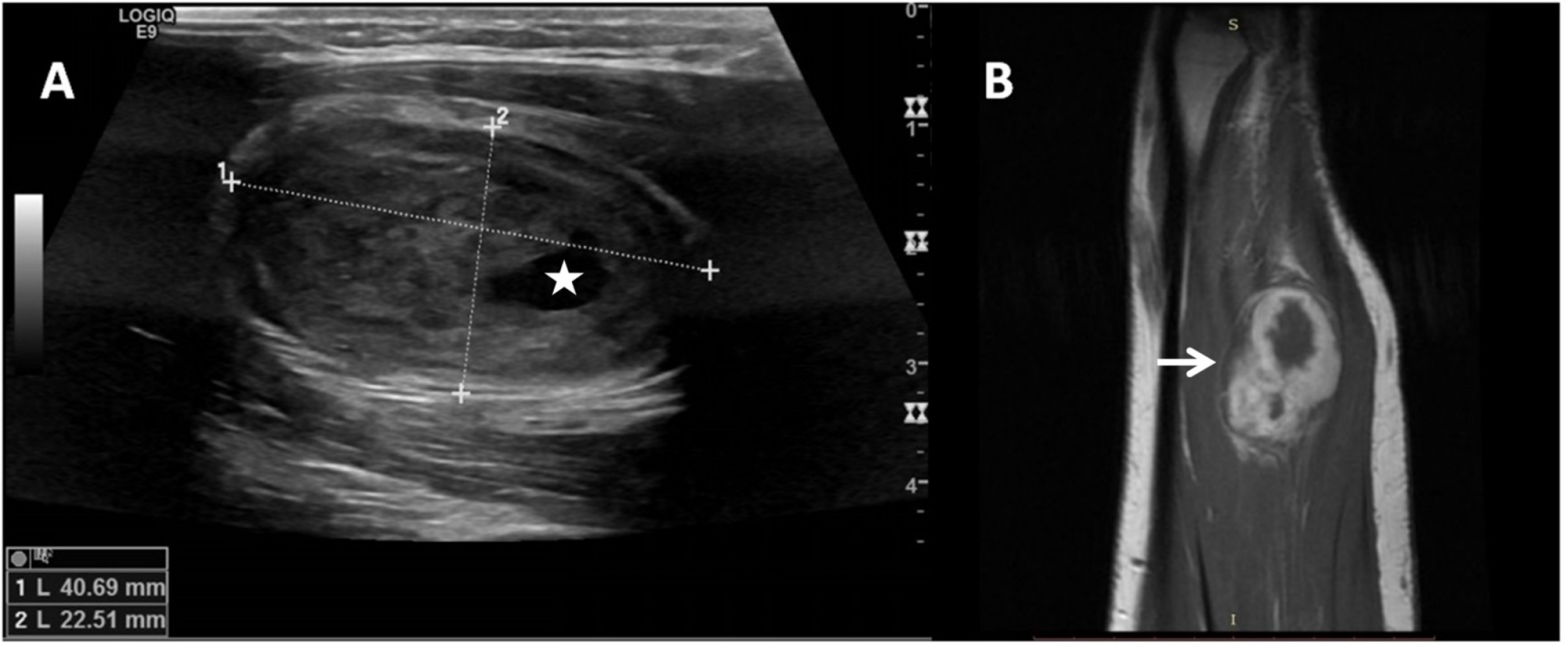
Ultrasonography and magnetic resonance images of the tumor (A) Ultrasonography revealed a hypoechoic solid mass with central cystic clarity (asterisk) in the proximal forearm. (B) Magnetic resonance imaging with contrast enhancement revealed a soft tissue mass (white arrow) on the median nerve route in the flexor muscles of the forearm.
